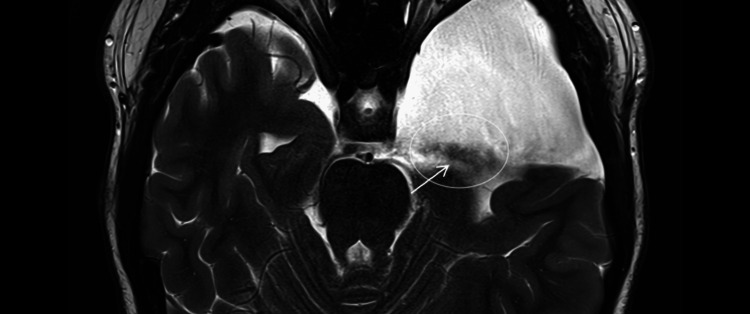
MRI (April 28, 2025; axial T2-weighted; 4-mm slices; 0.5-mm gap). Flow-related artifact along the medial wall of the left middle-fossa arachnoid cyst (arrow, open circle), supporting patency of the surgical fenestration and cyst-basal cistern communication.
